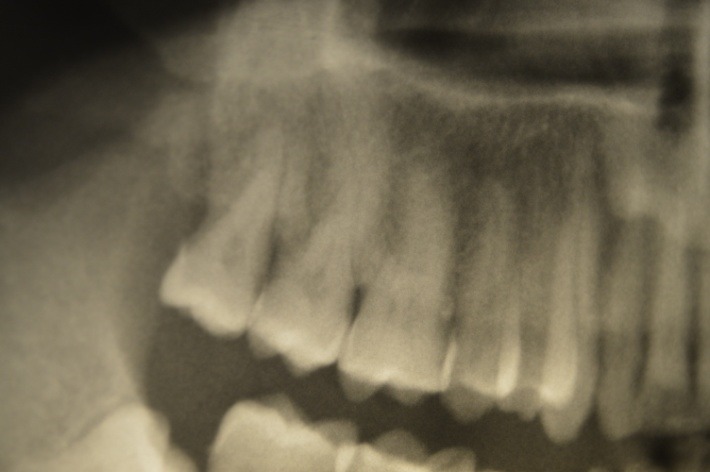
X-ray upper molars with pulp chamber modification
